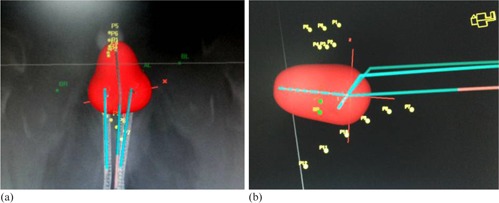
CT scan showing pear‐shaped isodose distribution on axial (a) and sagittal (b) images.
